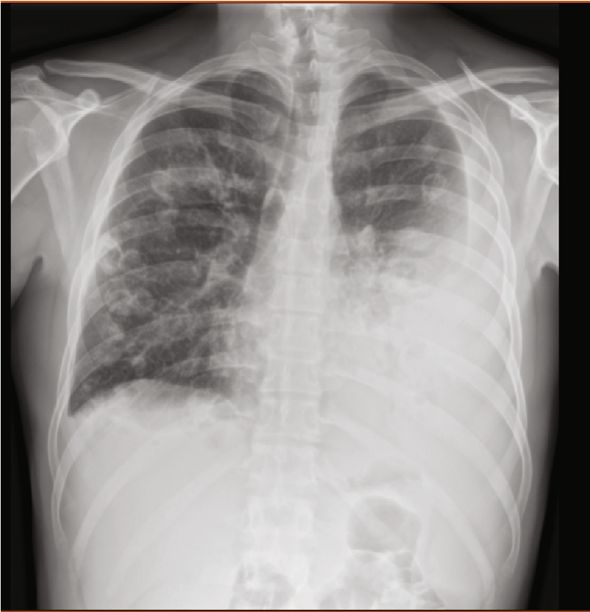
Chest x-ray with bilateral pulmonary nodules and pleural effusion.
